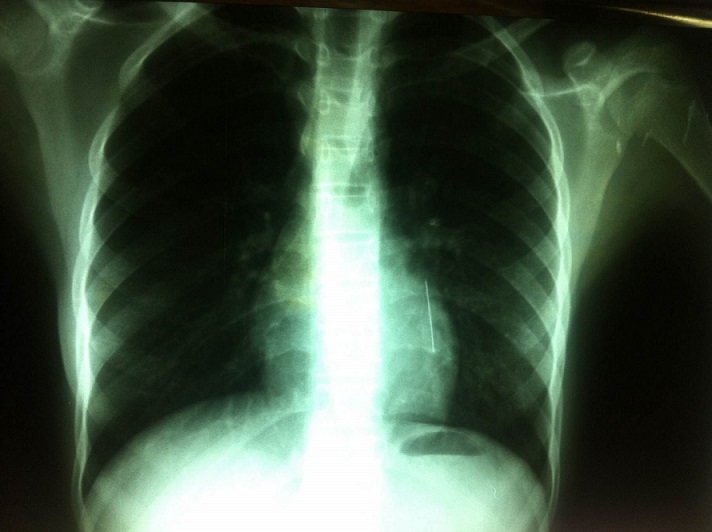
Radiographie thoracique de face montrant une épingle à foulard au niveau du segment paracardiaque gauche
